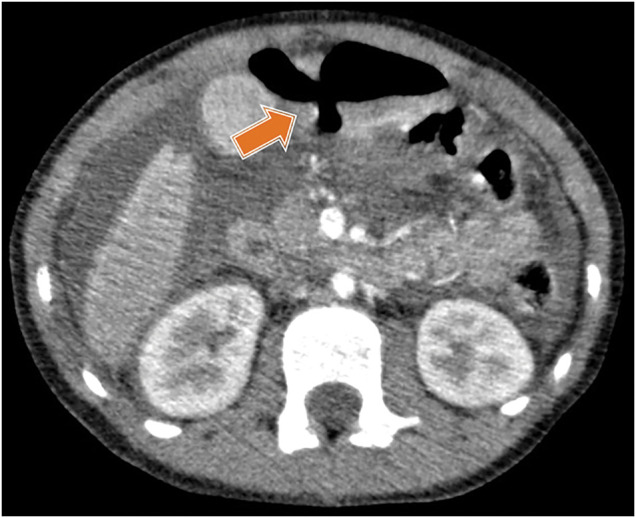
Contrast-enhanced CT 44 h after injury. Marked ascites and free air with an area of suspected injury were observed in the gastropyloric region region, which is indicated by the arrow.
